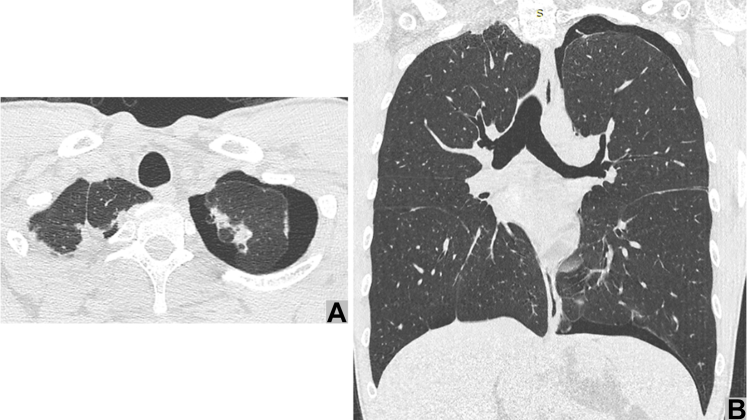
A, B, Chest high-resolution CT scan from initial presentation 3 y earlier: (A) axial and (B) coronal reconstructions showing bilateral apical pleural thickening with a persistent left-sided pneumothorax.
